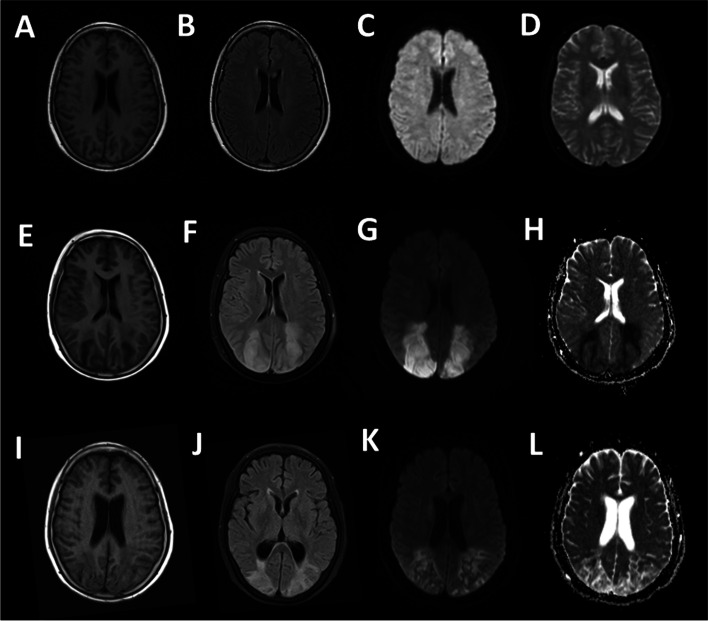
Initial and follow-up axial T1WI, FLAIR, DWI, and ADC brain MRI. A-D: Axial T1WI, FLAIR, DWI, and ADC brain MRI performed 5 days after inhalation indicated that there was no noticeable abnormality. E-H: Axial T1WI, FLAIR, DWI, and ADC brain MRI performed 12 days after exposure demonstrates extensive cytotoxic edema in the parietal and occipital lobes bilaterally, left frontal lobe, centrum semiovale region. I-L: Axial T1WI, FLAIR, DWI, and ADC brain MRI performed 59 days after exposure demonstrates a notable improvement of the intracranial arterial stenosis
